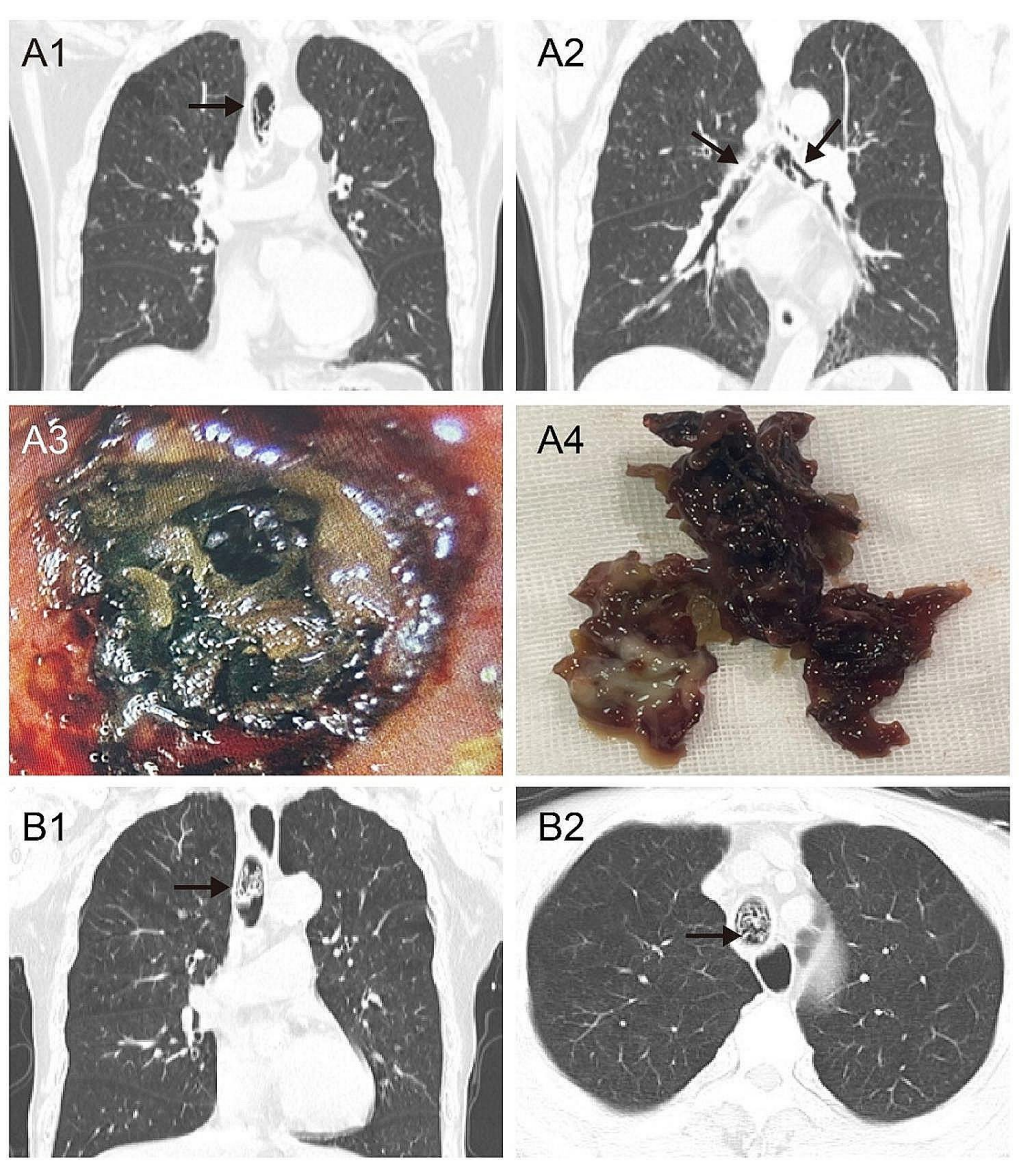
Sputum crust formation in patients receiving tracheostomies. Case 1 (A1-4). A 70-year-old male patient, 5 years after a total laryngectomy, was admitted with dyspnea for 2 days. The oropharyngeal and tracheal swabs were positive for *SARS-CoV-2.* After admission to the hospital, the patient underwent computed tomography (CT) scan of the lung and laryngoscope examination. A1: Dense shadow (indicated by black arrow) in the trachea as indicated by CT. A2: Dense shadow (indicated by black arrow) in the bronchus as indicated by CT. A3: Endoscopic manifestation of brown sputum crusts formation in the trachea causing airway obstruction. A4: Massive sputum crusts were removed from the airway by emergency surgery. Case 2 (B1-2). A 70-year-old male patient, 10 years after a total laryngectomy, was admitted with dyspnea for 3 days. B1-2: Dense shadow (indicated by black arrow) in the trachea as indicated by CT
